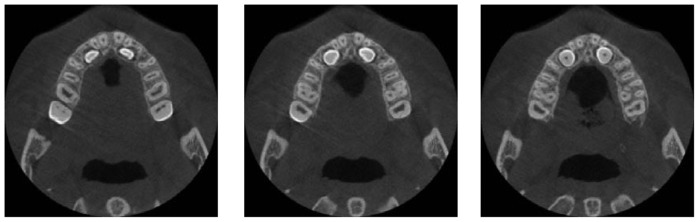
Three slices from the CBCT data set corresponding to the upper jaw.
